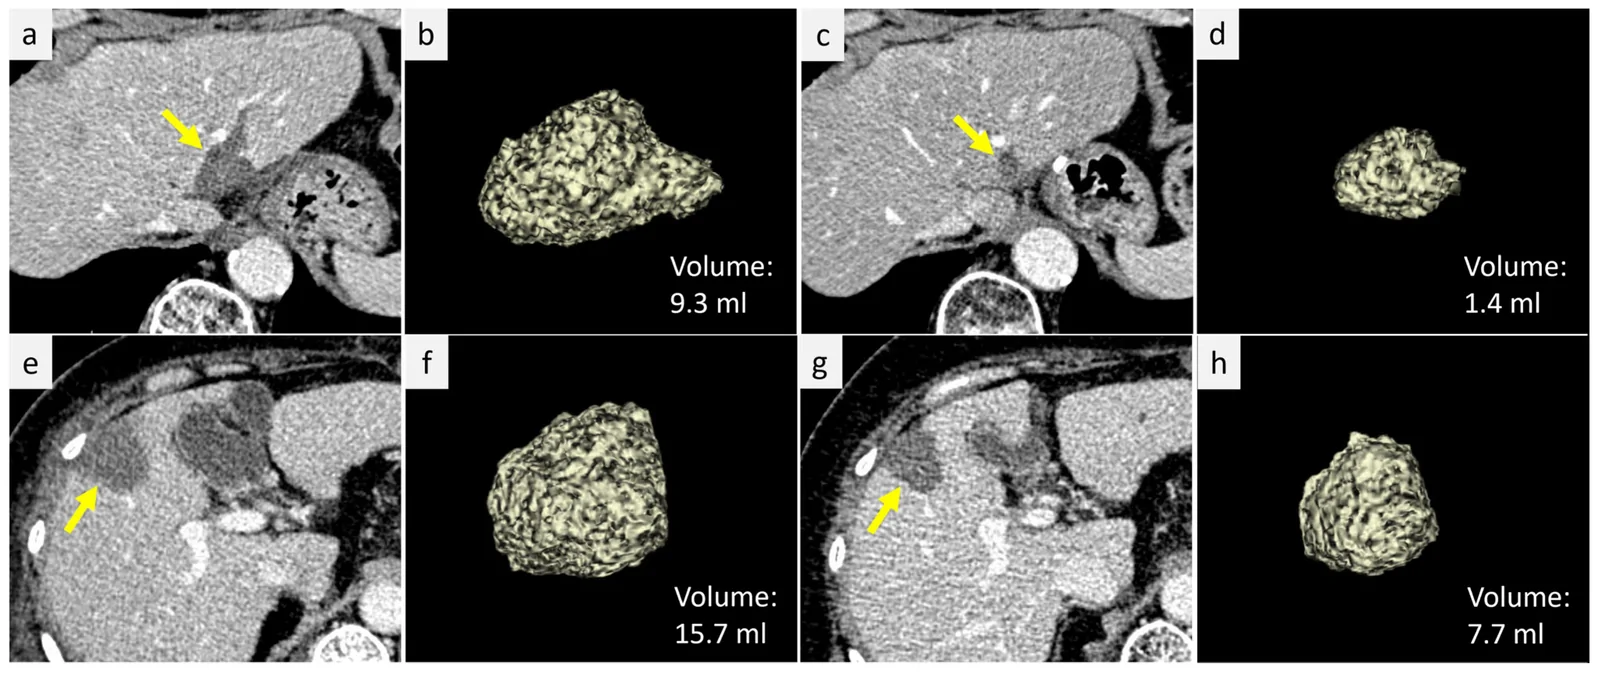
Representative images of the ablated area in the liver (yellow arrows): contrast-enhanced CT images obtained within 1 week (a,e) and around 6 months (c,g) after RFA and 3D images within 1 week (b,f) and around 6 months (d,h) after RFA. In a 72-year-old man with preserved liver function (Child-Pugh A and ALBI score of −2.59/mALBI grade 2a) (a–d), the reduction rate of AAV was 84.6%. In an 81-year-old woman with impaired liver function (Child-Pugh B and ALBI score of −1.46/mALBI grade 2b) (e–h), the reduction rate of AAV was 51.0%.
